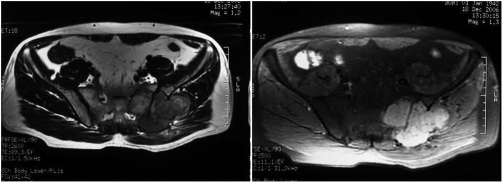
Pre-therapy CT images. (A) Axial T2-weighed image of the pelvis: shows a large lobulated mass with intermediate signal intensity. (B) Post contrast T1W Fat Sat image: shows intense enhancement of the mass.
